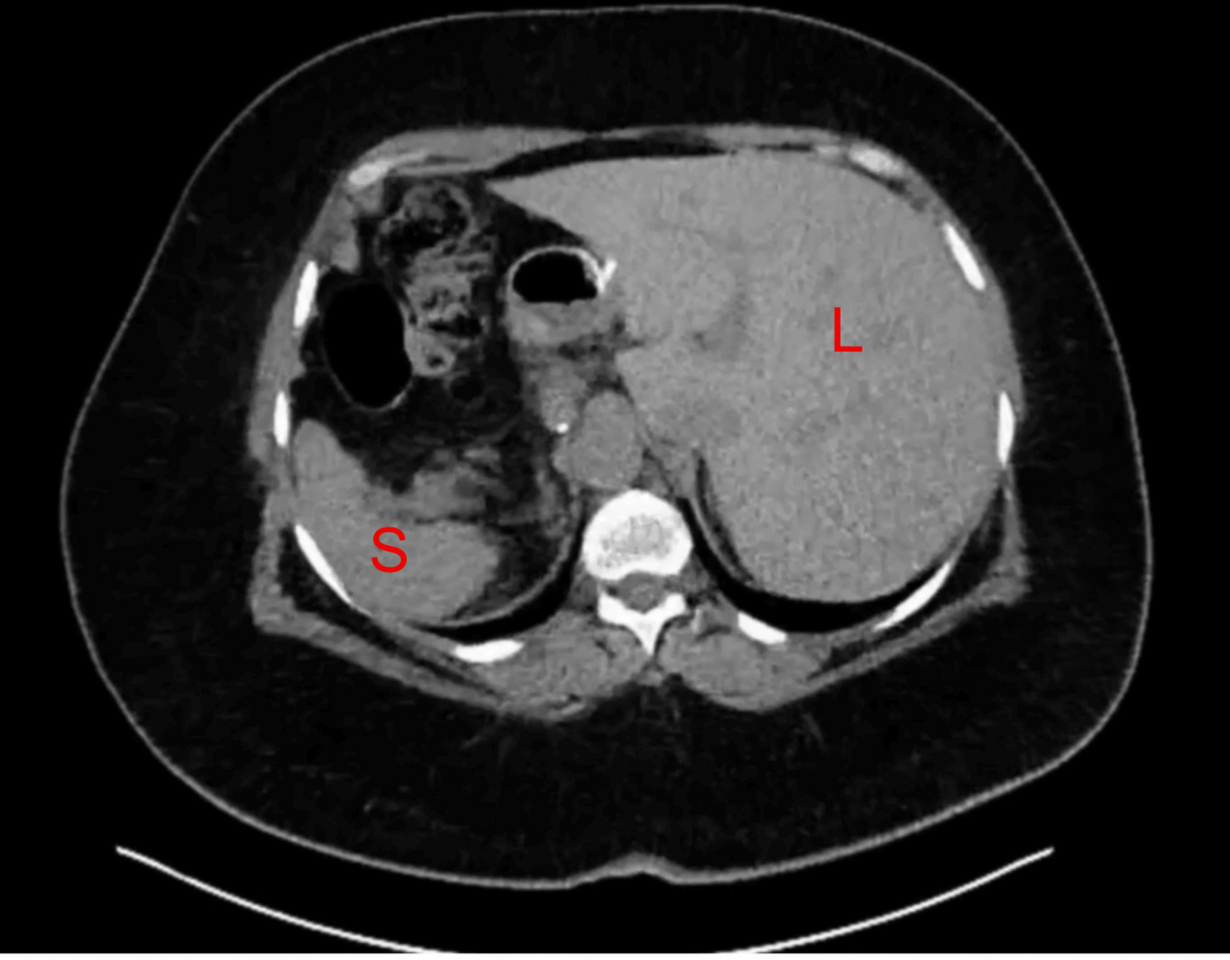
Abdominal CT scan shows organs in opposite anatomical positions Liver (L) seen on the left side of the body and spleen (S) on the right side of the body.
